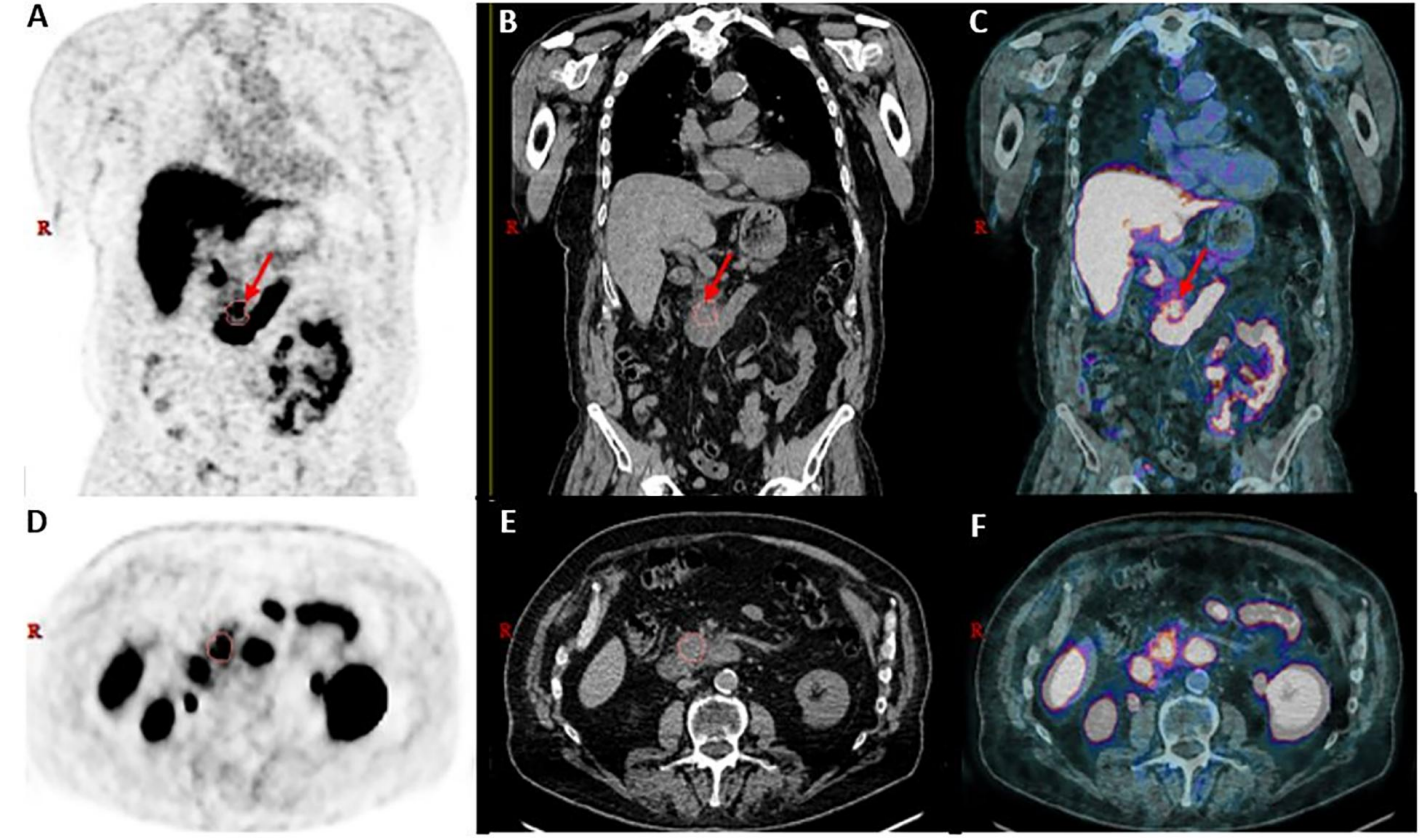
18F-DCFPyL PET/CT images showing uptake in the pancreatic uncinate process (orange borders/red arrow). (A–C) Coronal PET, CT, and fused PET/CT. (D–F) Transaxial PET, CT, and fused PET/CT.
